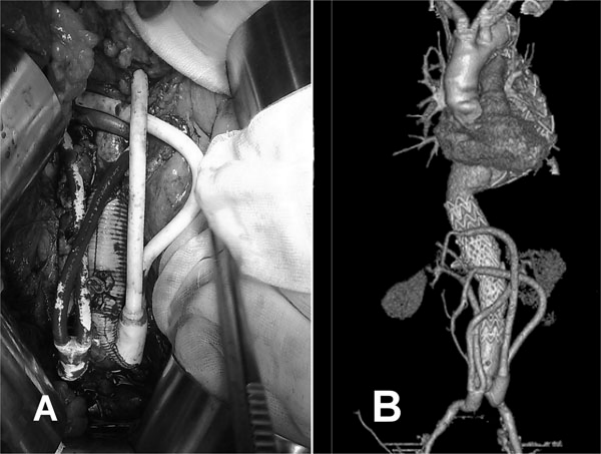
(A) Intraoperative view. (B) Early angio CT scan showing the implanted stent-grafts and the patency of all prosthetic bypass.
